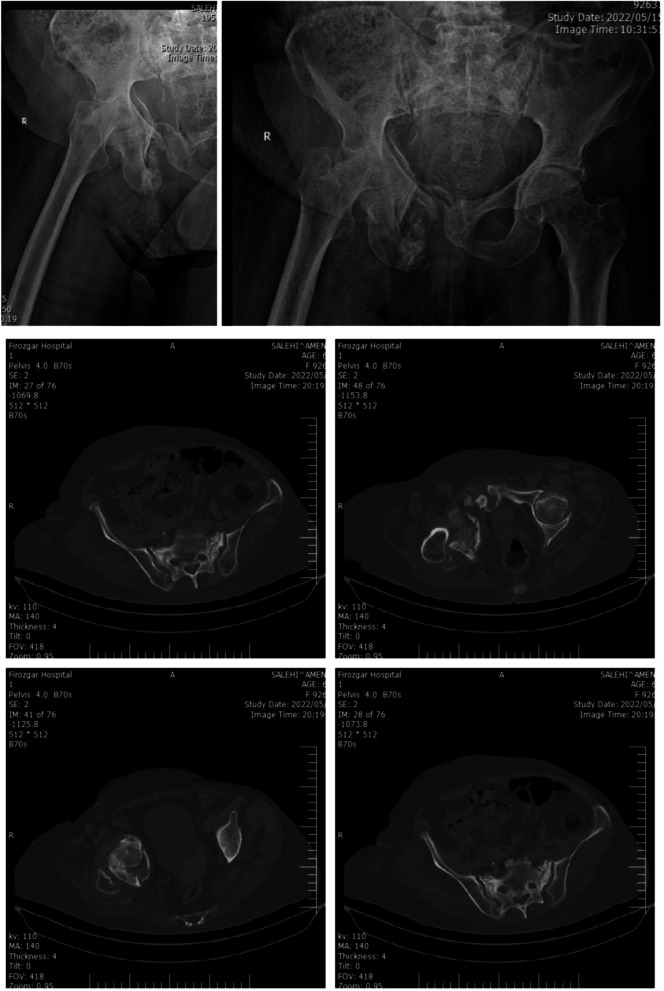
Patient’s pre operation radiographs
